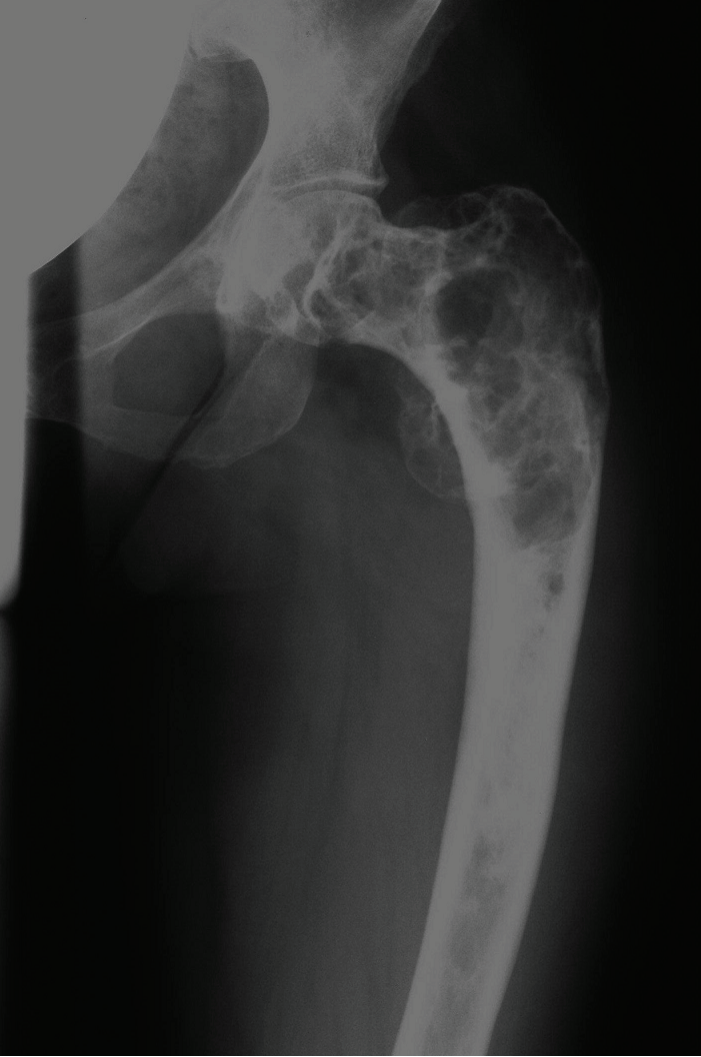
X-ray of the left femur. Anteroposterior plain film of the upper left leg with typical osseous changes consistent with fibrous dysplasia and shepherd's crook deformity.
